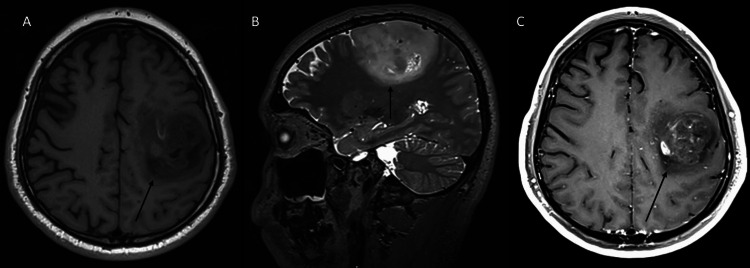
Preoperative cranial MRI (May 2021). (A) T1-weighted cranial MRI, axial section; (B) T2-weighted cranial MRI, sagittal section; (C) with-contrast cranial MRI, axial section. An intra-axial lesion seen in the frontoparietal region, with involvement of the precentral and postcentral gyri; a heterogeneous lesion is identified in all sequences, with some hyperintense areas in T1; discrete and heterogeneous enhancement is observed with contrast medium. MRI: magnetic resonance imaging
